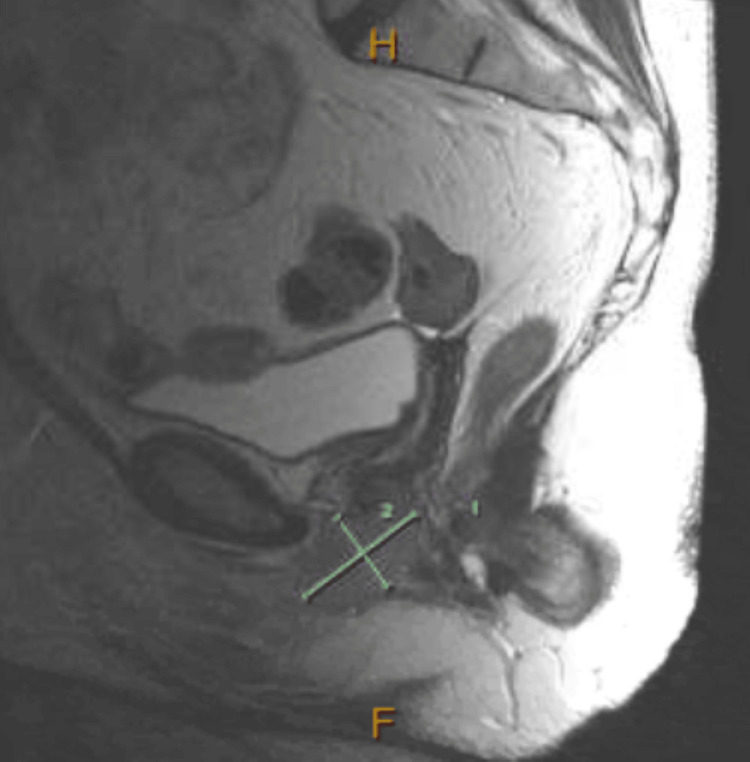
Sagittal section of Pelvic MRI demonstrating the vaginal carcinoma measuring 4.3 cm x 2.7 cm.
